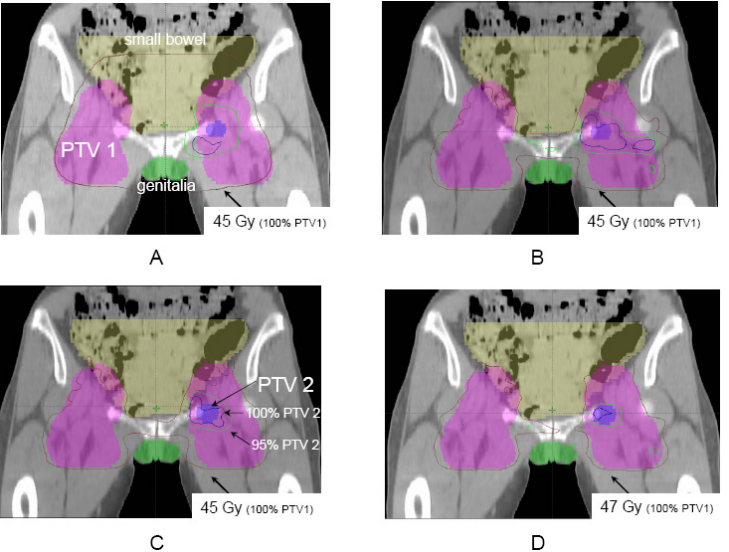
Dose distributions by treatment arm on the same coronal CT slice through both target volumes and avoidance structures. This CT slice also shows the sparing of the external genitalia, small bowel and iliac crests in arms B, C, and D as compared with arm A.
